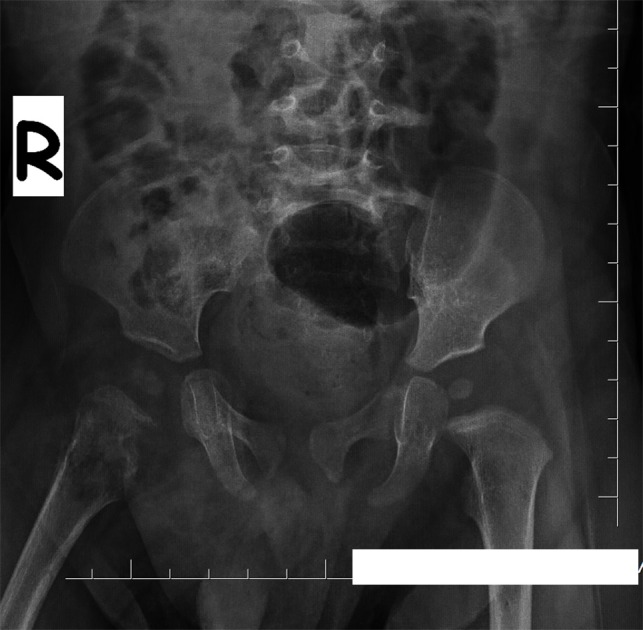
Figure 1: Magnetic resonance imaging (MRI) revealed a heterogeneous contrasting lesion on upper right femur: hypointense on T1 and hyperintense on T2 weighted images
